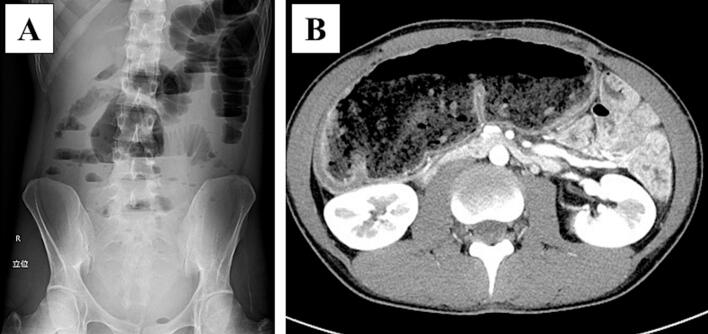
Preoperative findings of total colectomy. A Preoperative abdominal X-ray showed niveau formation. B Preoperative abdominal CT scan showed significant dilation of cecum to transverse colon.
